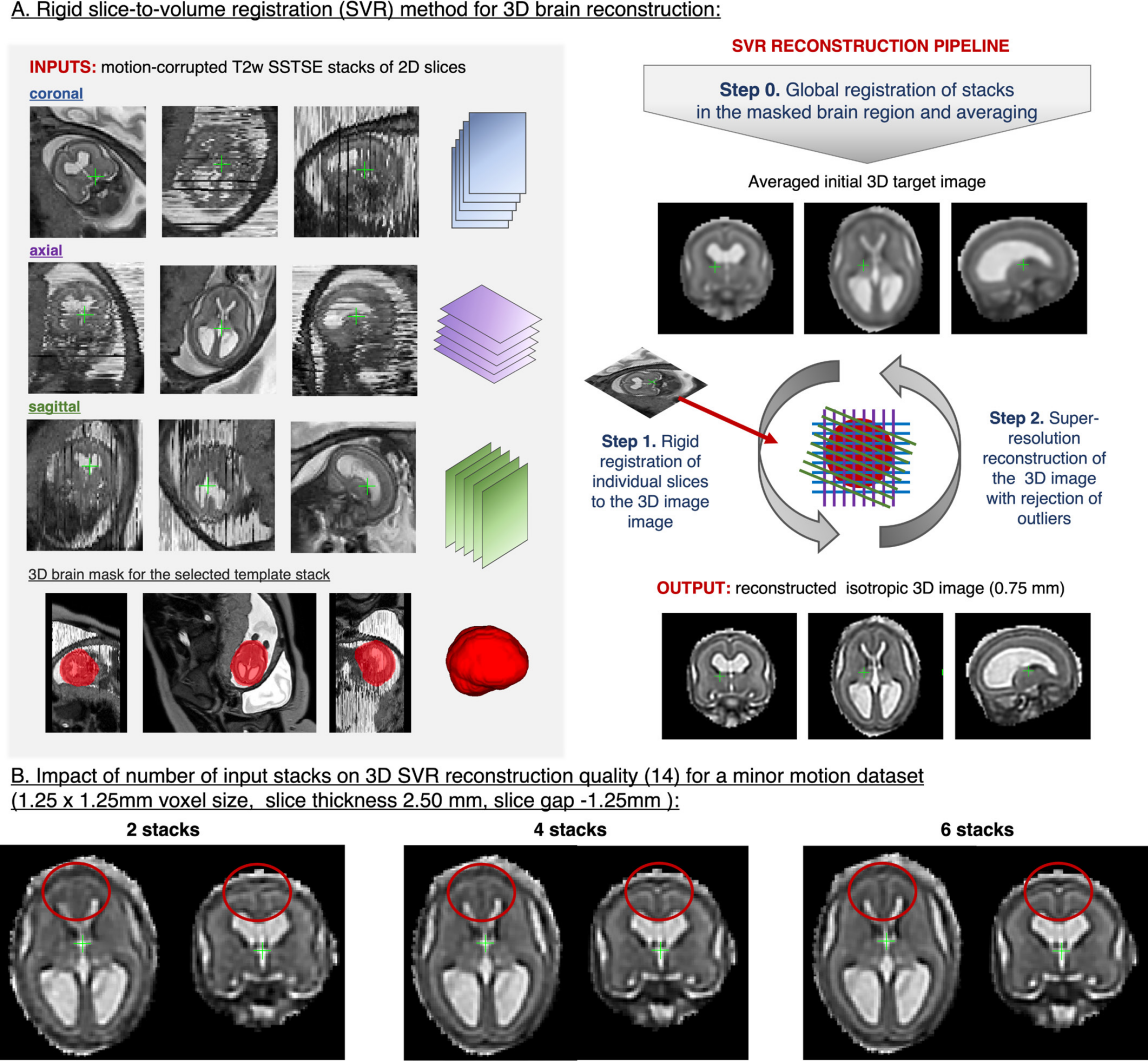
3D SVR reconstruction for foetal brain MRI. This example is based on the MRI dataset acquired at St.Thomas’ Hospital, London and reconstructed using the classical SVR. SVR, slice-to-volume registration
